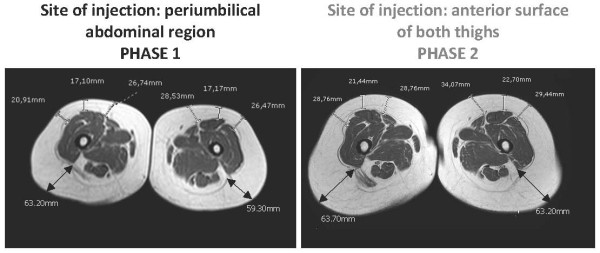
MRI scans of the thighs of patient 2 when injecting pegvisomant (10 mg/day) subcutaneously in her abdomen (Phase 1) and thighs (Phase 2).
